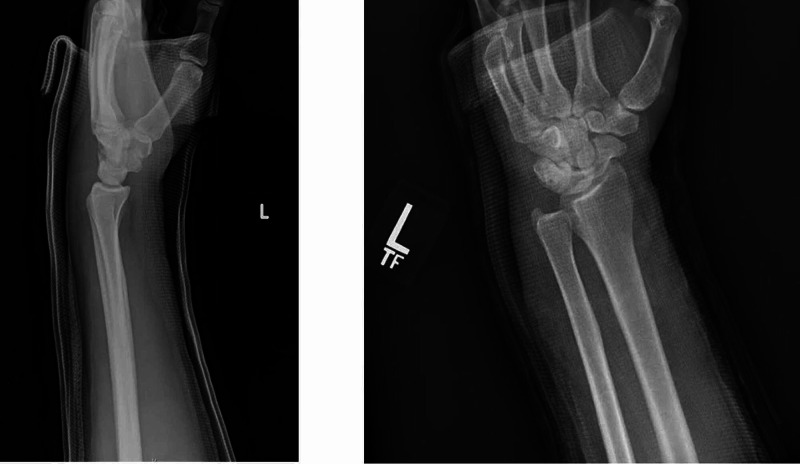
Post-reduction radiographs including lateral (left) and posteroanterior (right) views
